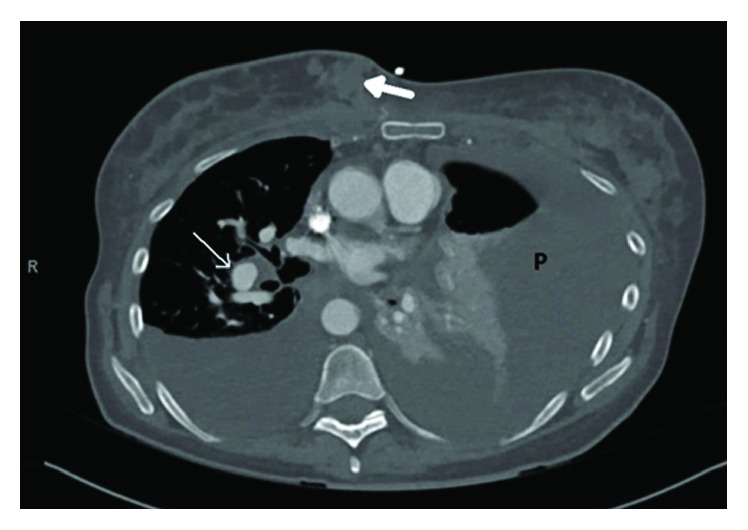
Computed tomography with angiography showing a right medial breast mass (closed white arrow) with mediastinal (open white arrow) and axillary lymphadenopathy. Large effusions greater on the left (P).
